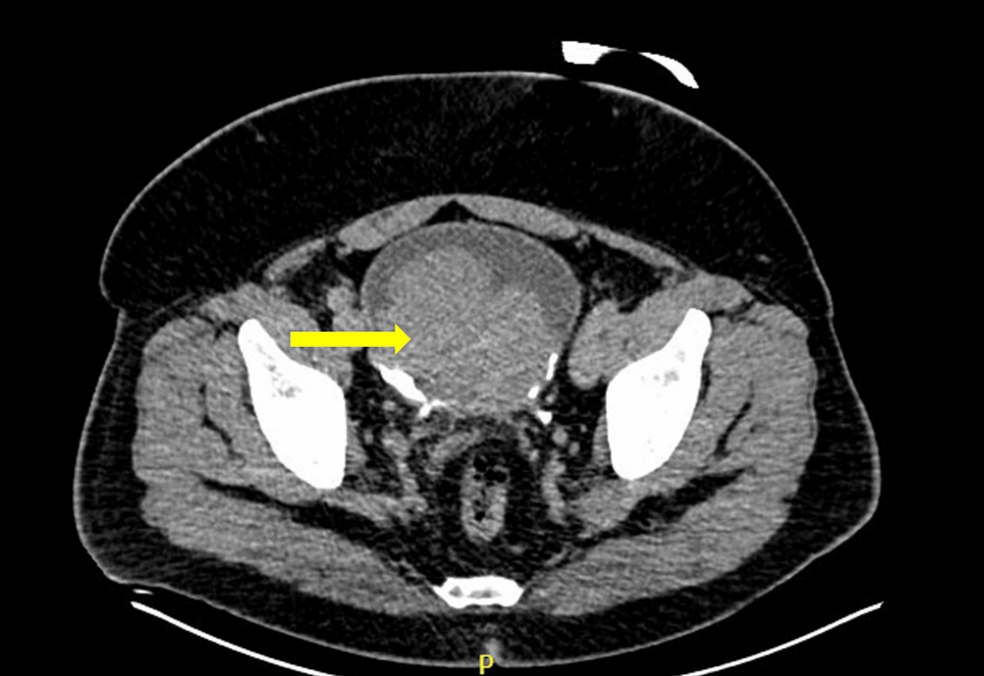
CT urogram with a large tumor burden (yellow arrow) within the bladder
